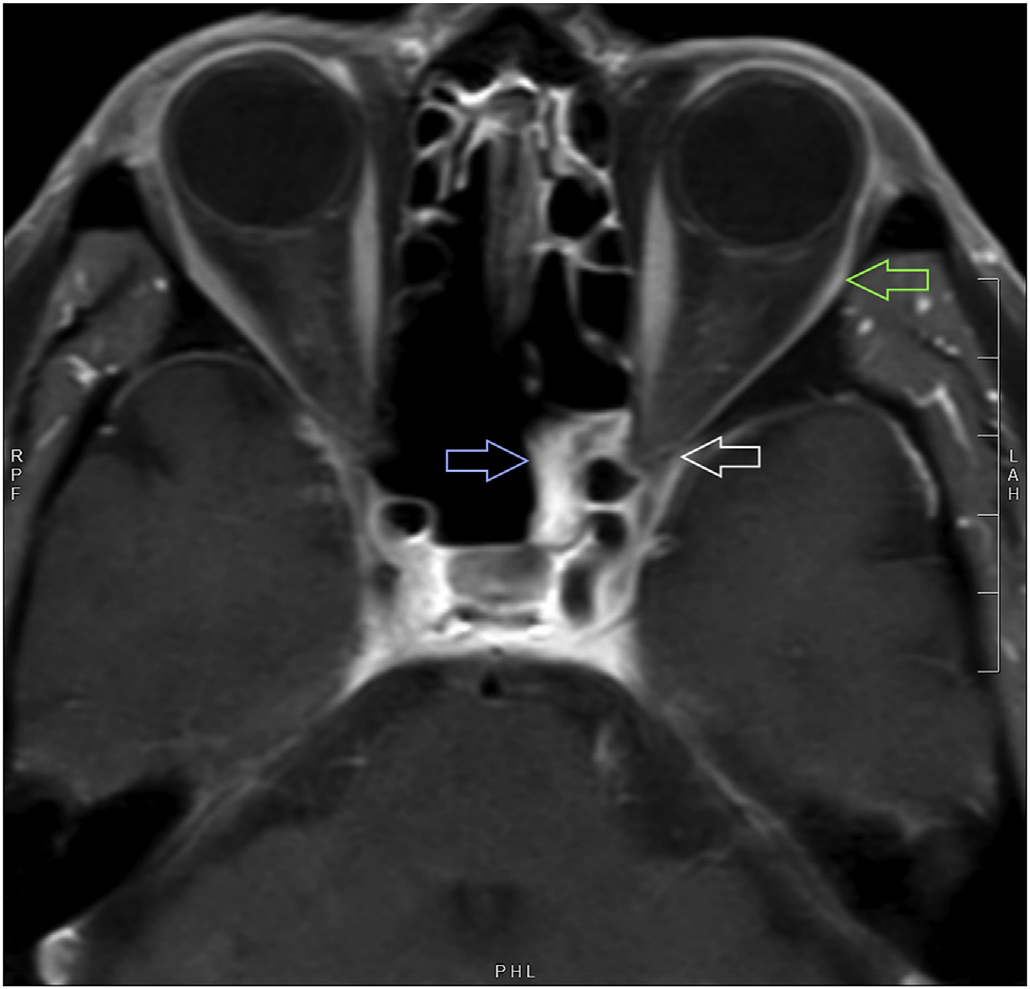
Magnetic resonance imaging. A T1-weighted, post-gadolinium, axial magnetic resonance imaging section of the brain and orbit showing left lateral rectus atrophy (green arrow), left sphenoid sinus opacification (blue arrow), and equivocal fullness of the superior orbital fissure (white arrow). (For interpretation of the references to color in this figure legend, the reader is referred to the Web version of this article.)
